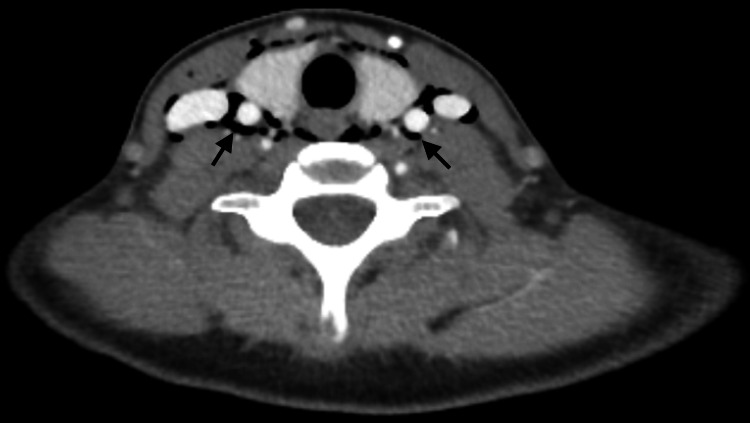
Computed tomography of the neck demonstrating extensive retropharyngeal, visceral, and carotid space emphysema
